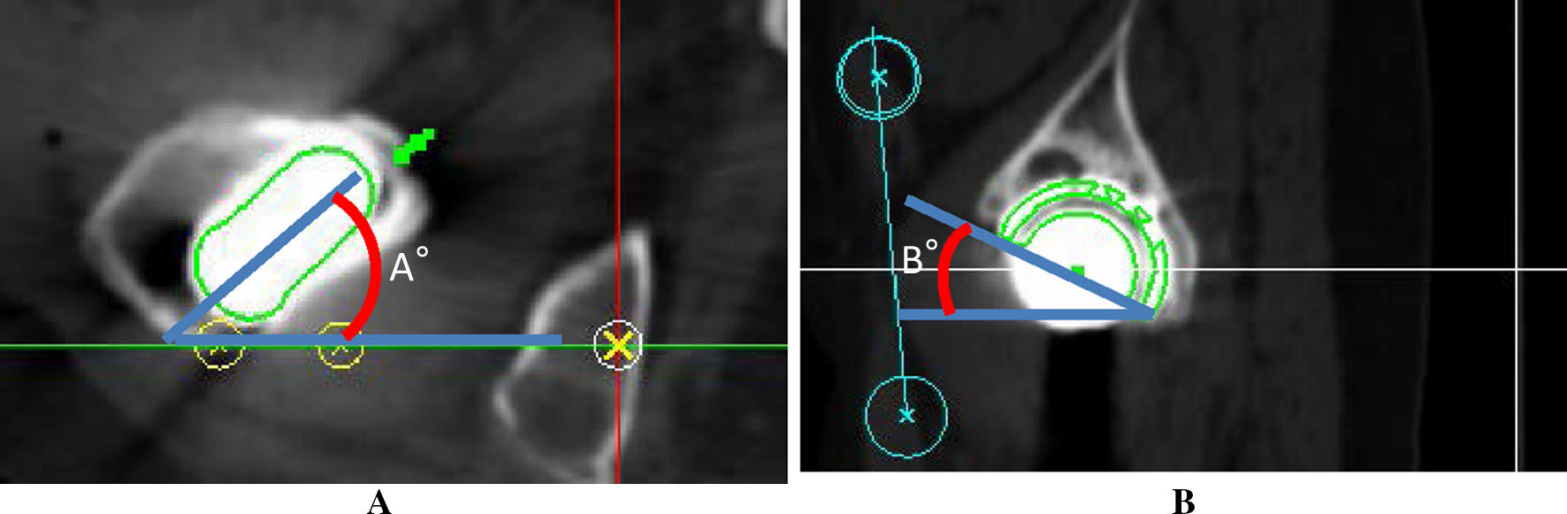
Combined anteversion = *A* + sin^−1^{sin (*B*) × cos (operative inclination)}. *A* = anteversion of the stem. *B* = operative anteversion of the socket. To obtain the socket anteversion angle, we measured the angle that the functional pelvic plane makes with the socket and converted the values into radiographic anteversion angle using the conversion equation described by Murray
